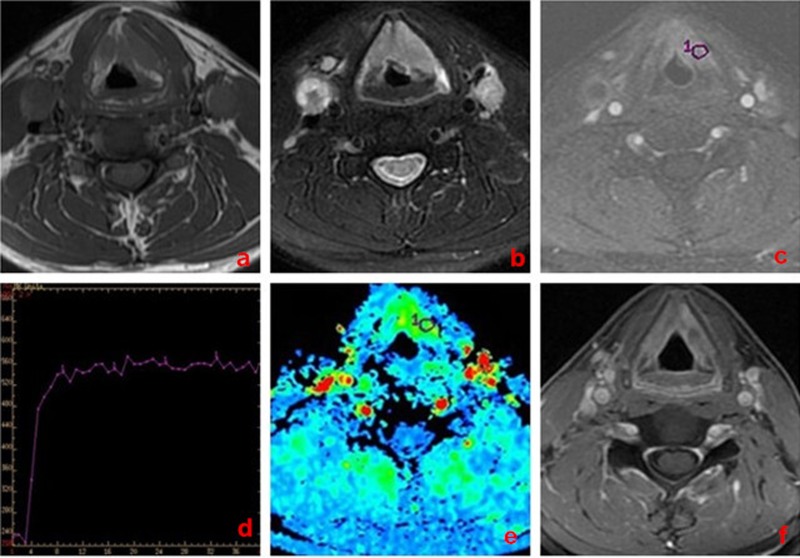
A 58-year-old man with larynx carcinoma, good response to chemo-radiotherapy a. Transverse T_1_-weighted imaging. b. Transverse T_2_-weighted imaging. c. DCE-MRI map. d. TIC map. e.The MSI map, MSI value was calculated as 168.74. f. Transverse T_1_-weighted enhancement imaging show that tumor mass was reduced obviously (> 50% reduction) after treatment.
